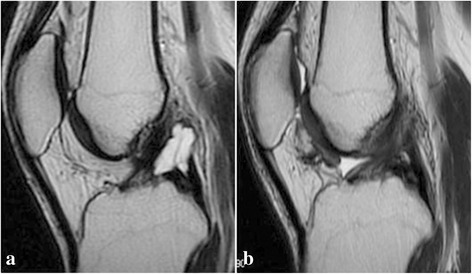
The preoperative and postoperative MRI images on a sagittal plane. a A multilocular PCL cyst. b There was no cyst recurrence at the final follow-up after operation
